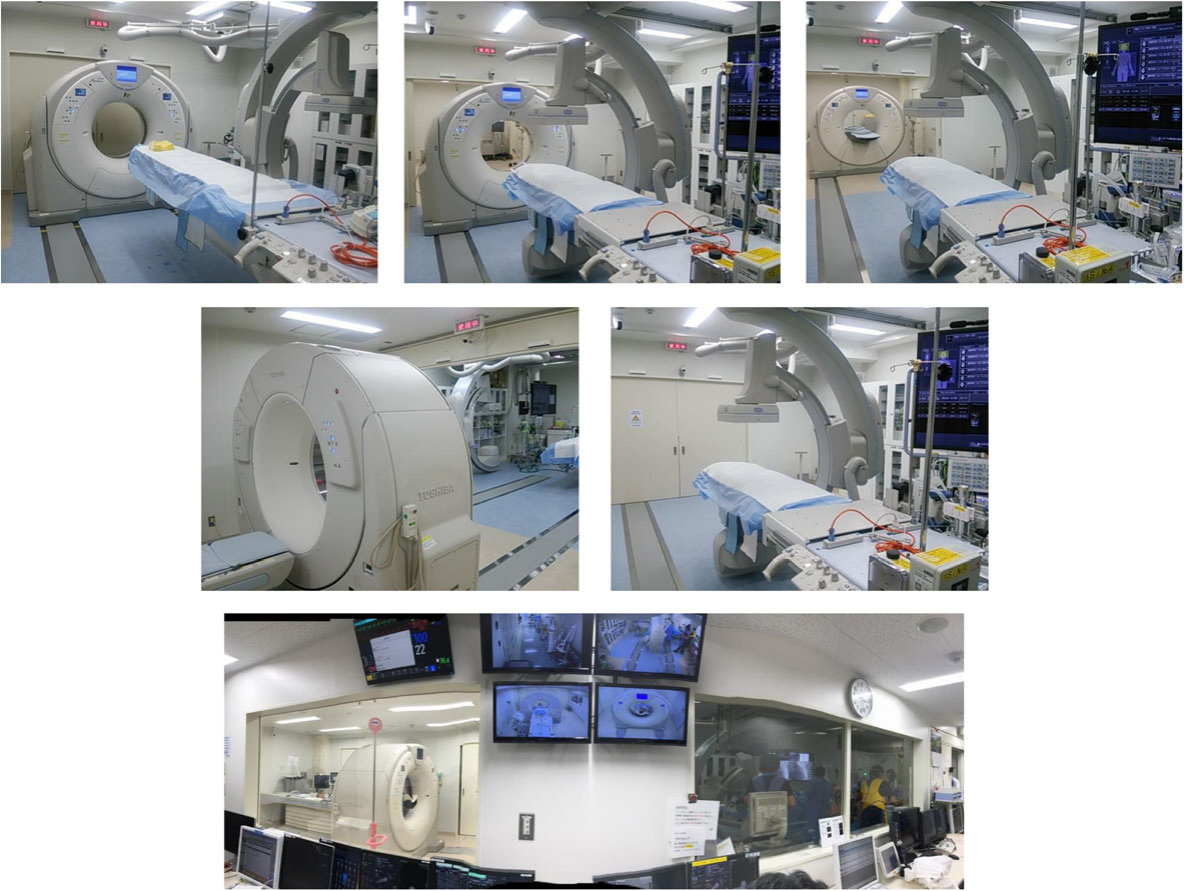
Photographs showing the dual-room sliding CT scanner system with interventional radiology features. The new CT suite has another radiolucent table. When we perform emergency surgery or interventional radiology for a severely injured or ill patient in the regular emergency room, the sliding CT scanner is moved to the new adjacent CT suite with the radiolucent table, and we can perform CT scanning of another in/outpatient
